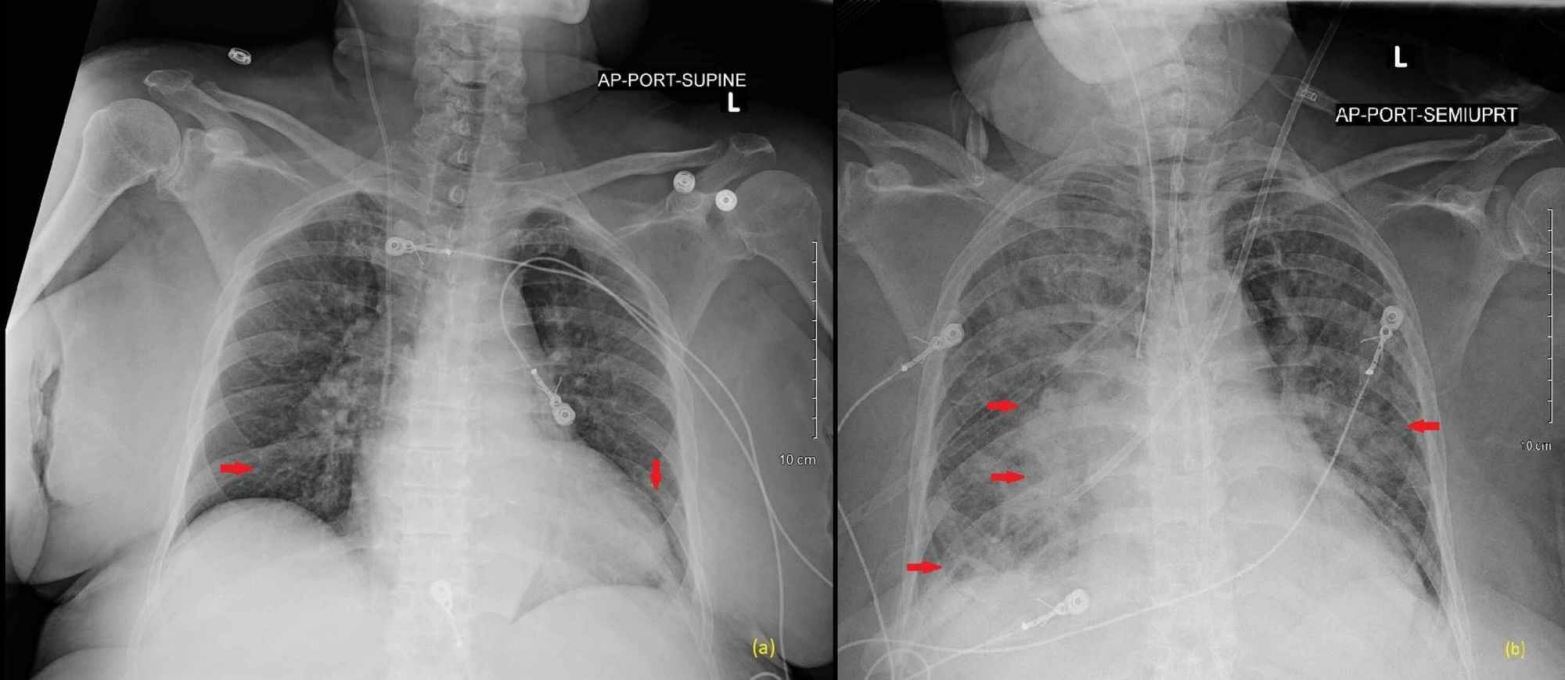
Portable chest radiograph (a) X-ray on admission showing low lung volumes and bibasilar atelectasis; (b) follow up X-ray on day 15 showing diffuse bilateral multifocal opacities greater in the right lung consistent with pneumonia.
